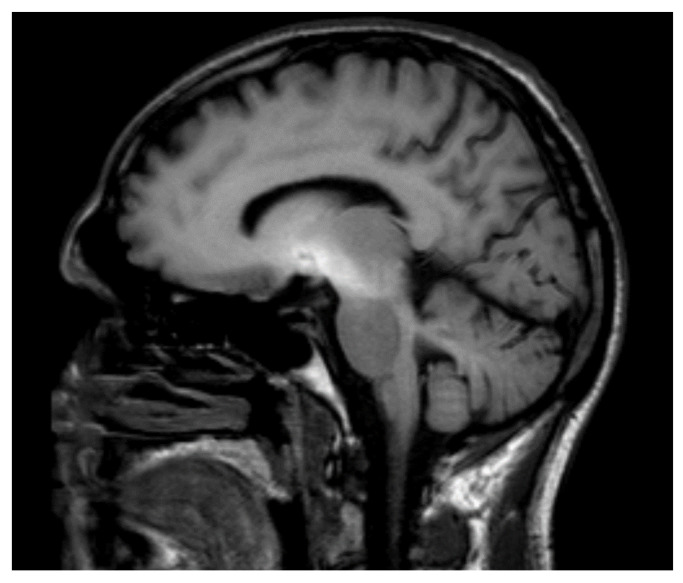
MRI T1 basal ganglia in intravenous methcathinone users. Nonenhanced, T1 weighted MR images show markedly hyperintense signals in the medial and lateral part of the globus pallidus, putamen, and in a lesser extend in part of thalamic nuclei.
